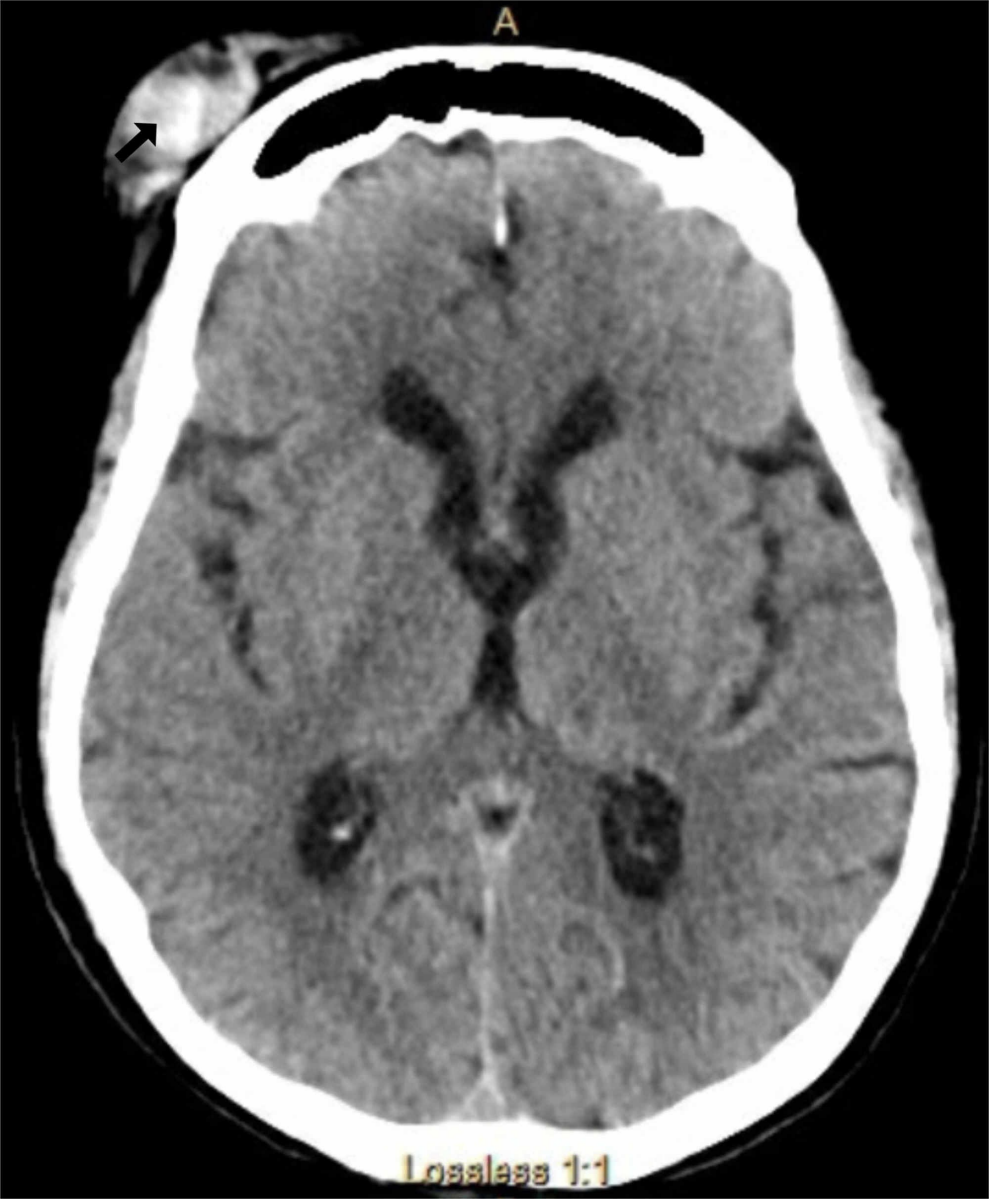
A CT image of the head demonstrates an extra-axial hematoma overlying the frontal bone. This finding is not relevant to the case report, as a hematoma cannot cause an oculogyric crisis.
